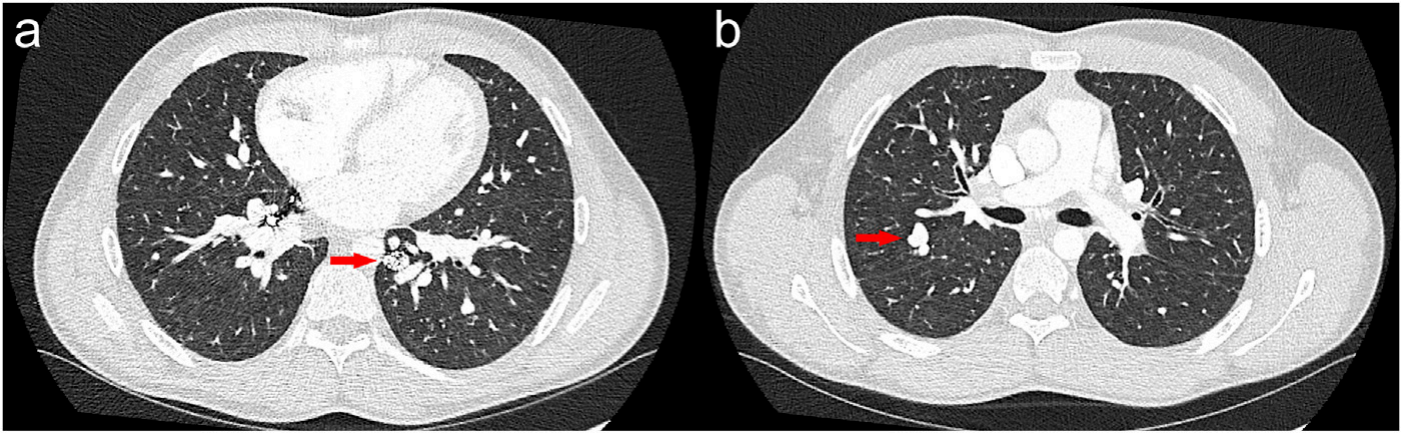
Axial computed tomography imaging of the chest in patient II-5, demonstrating diffuse bilateral pulmonary arteriovenous malformations as well as several embolization coils in the bilateral lower lobes and right middle lobe. There is persistent flow distal to many of the embolized arteriovenous malformations. (A) Example of an untreated pulmonary arteriovenous malformation in the posterior right upper lobe measuring 1.2 cm in all dimensions and (B) a case of a previously embolized arteriovenous malformation in the left lower lobe measuring 1.5 × 2.1 × 1.7 cm.
